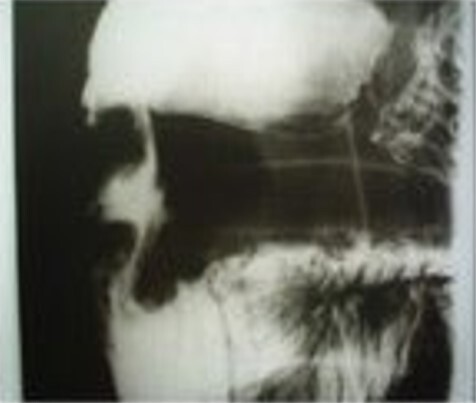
Contrast radiographs showing stenosis of the second portion of the duodenum in a patient with groove pancreatitis who underwent emergency gastroenteroanastomosis.
